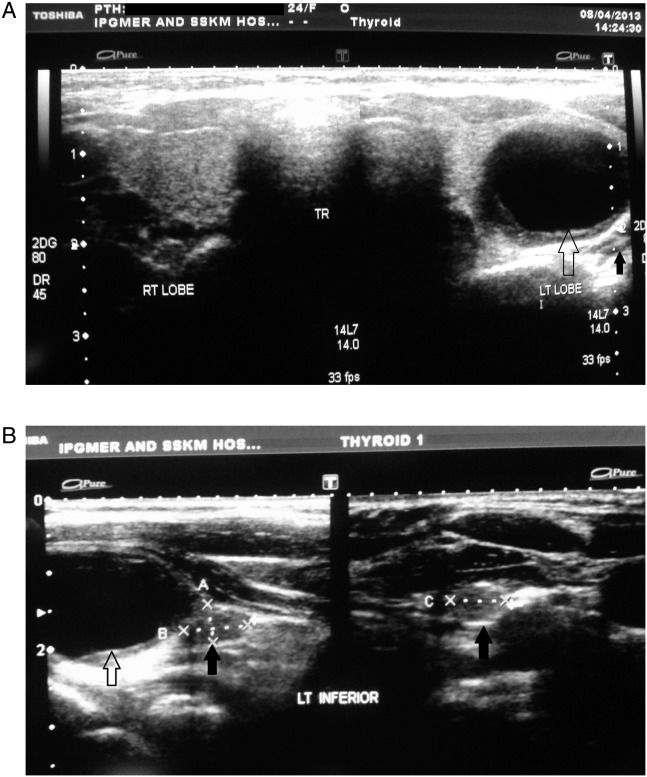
(A) Ultrasonography of the neck revealed simple cyst in the thyroid parenchyma corresponding to the palpable nodule in the left lobe (hollow black arrow). A 0.6×1.0 cm hypoechoic well-demarcated elliptic lesion with smooth borders and a hyperechoic line on the ventral surface were also noted posterior to thyroid (solid black arrow). (B) Ultrasonography neck with better characterization of the hypoechoic elliptic lesion posterior to left lobe thyroid suggestive of parathyroid adenoma (solid black arrow). The intrathyroidal simple cyst can be noted adjacent to it (hollow black arrow).
